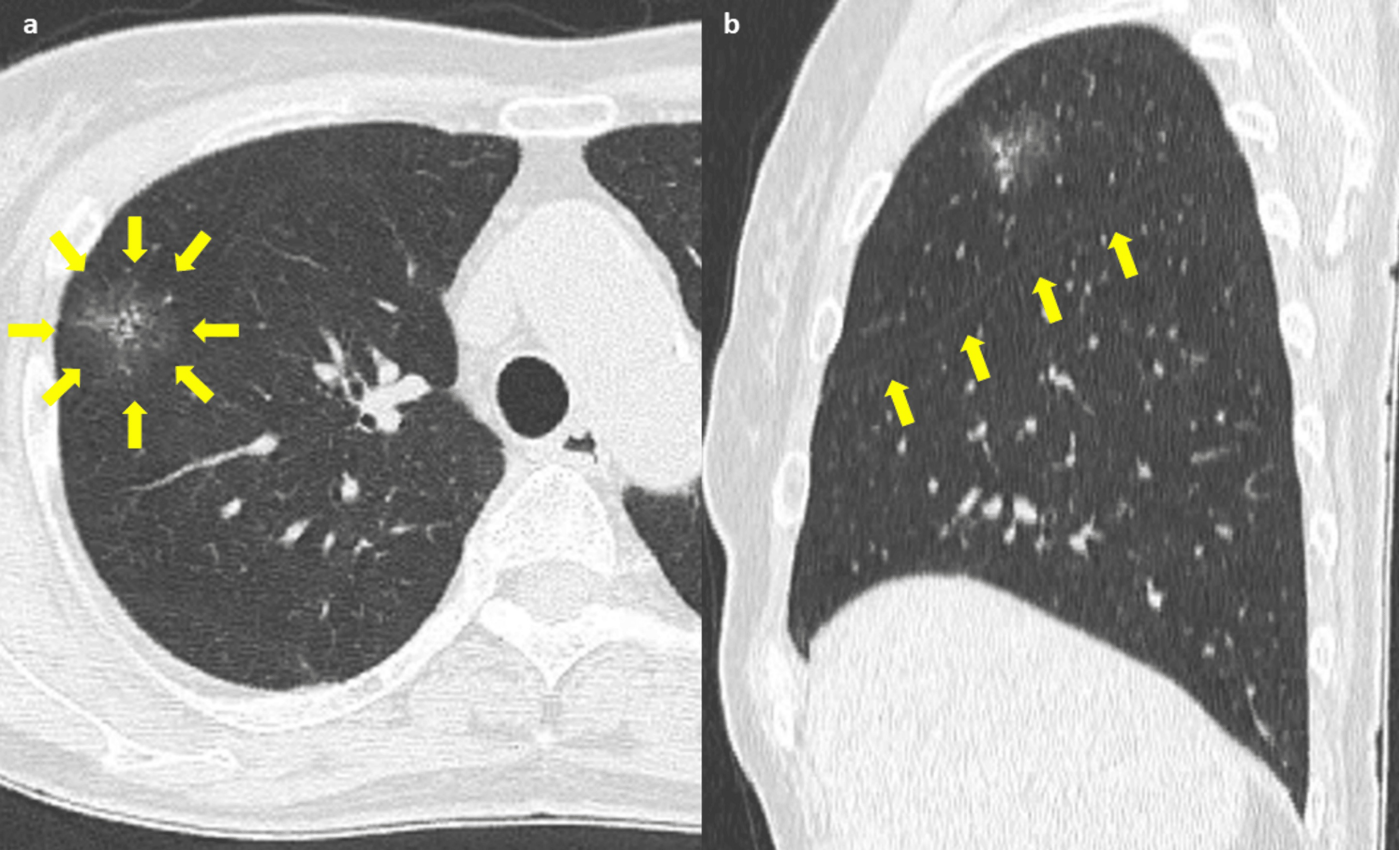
Chest computed tomography images (axial and sagittal views) (a) A mixed ground-glass nodule, 2.0 cm and 0.7cm in total and solid size, located between the right upper and middle lobes. (b) Yellow arrows indicate the major fissure. The minor fissure is barely visible.
